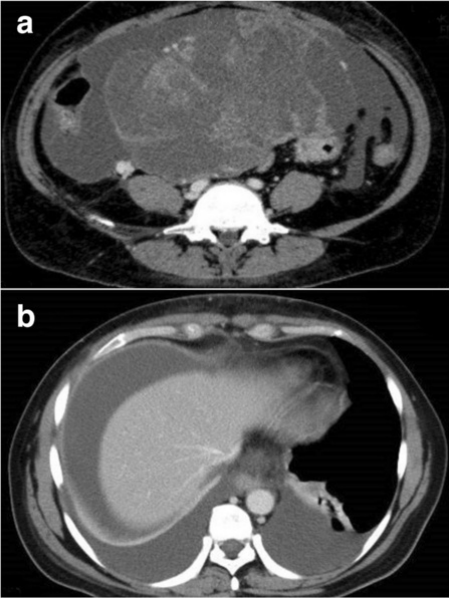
The second computed tomographic scan, obtained 2 weeks after the initial scan, demonstrated massive ascites (a) and bilateral pleural effusion (b)
